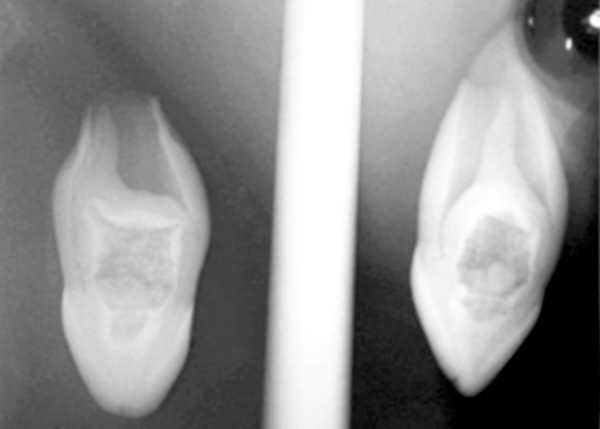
Periapical radiograph (labial and mesial views) of 22 showing a type III dens invaginatus, a main canal, pseudo canal, invagination and a large pulp stone
